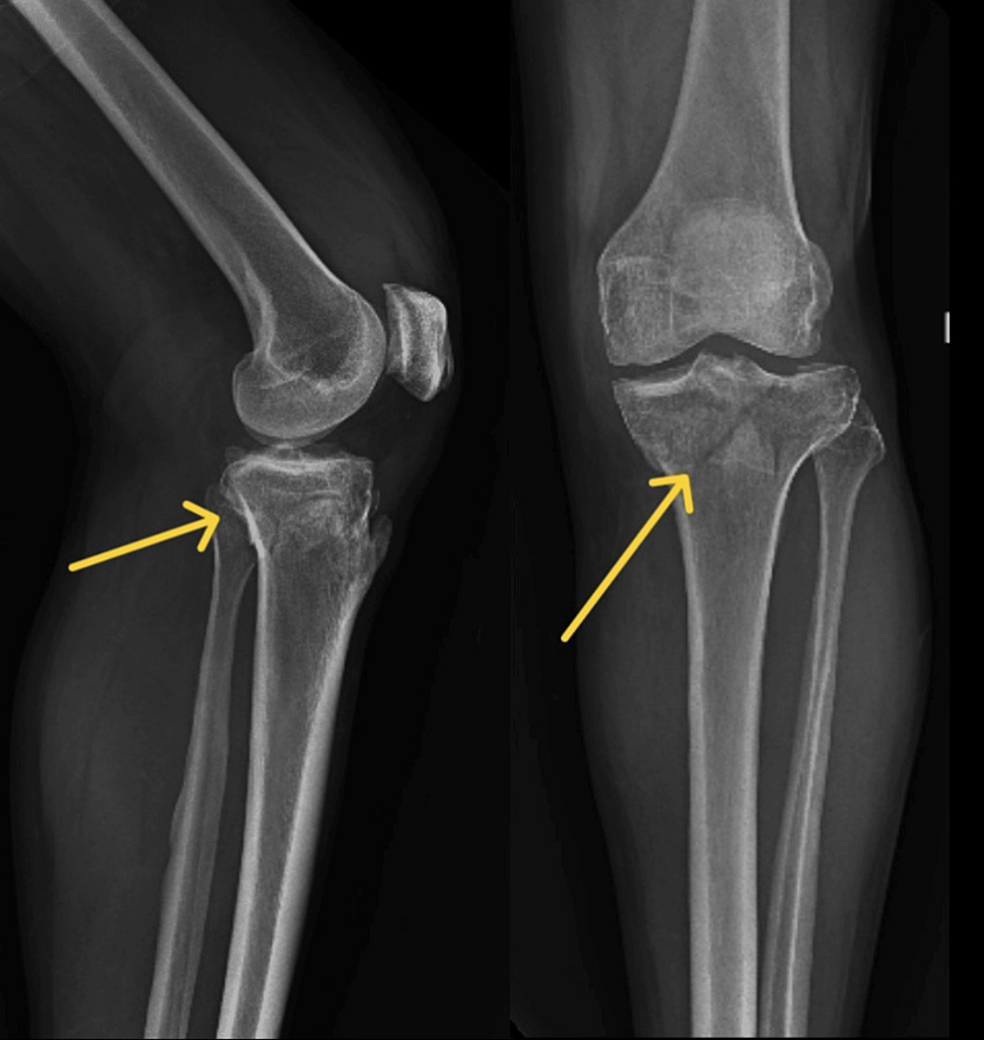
The pre-operative radiograph of the left knee joint lateral and anteroposterior views
